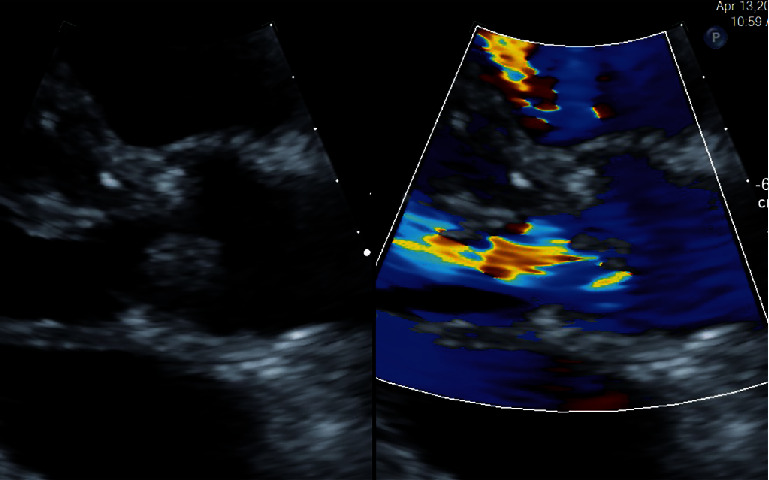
TTE from parasternal long axis view showing large echodensity on the right coronary cusp. Color Doppler demonstrates AI.
